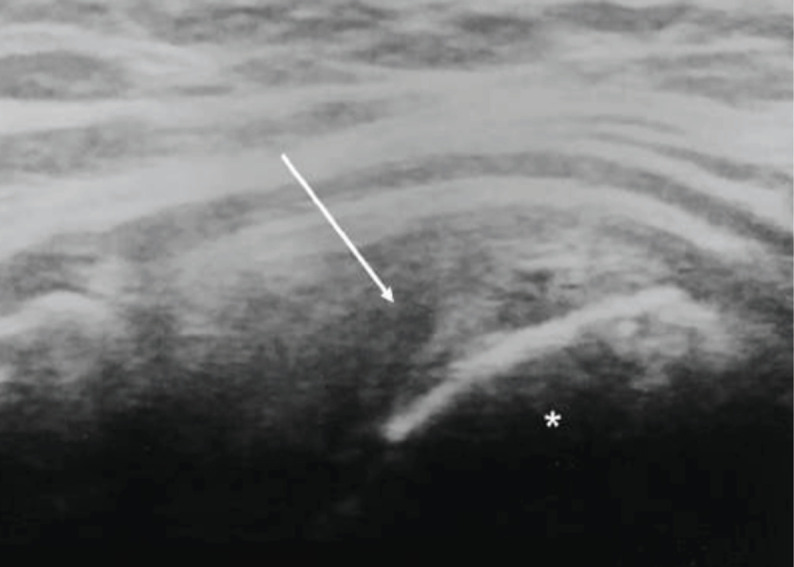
Ultrasonographic evaluation of full-thickness tear of supraspinatus tendon. (Arrow: tendon defect, Asterisk: greater tuberosity).
